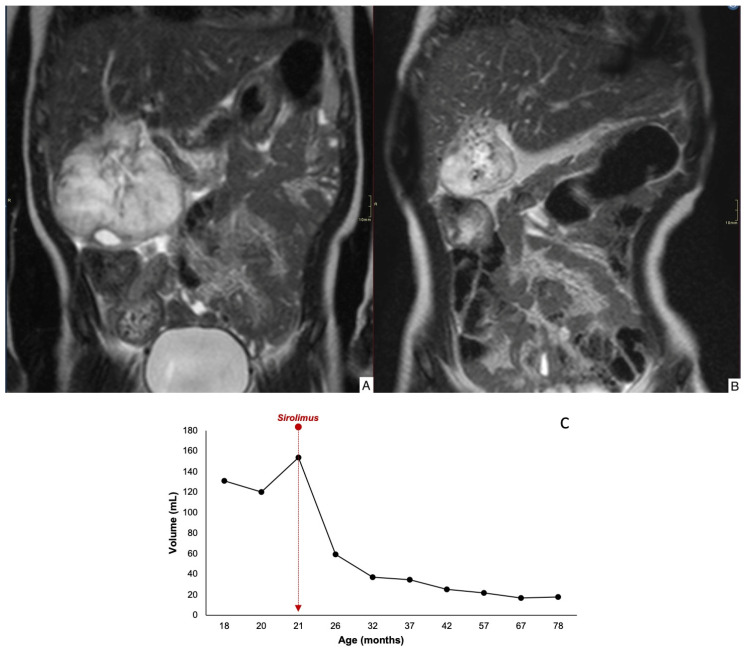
Coronal T2-weighted MRI image (T2WI) through the abdomen of an 1-yr-old girl depicts an exophytic and T2-hyperintense mass in the liver (A). Coronal T2WI after 18 months of treatment with sirolimus, the mass has markedly decreased in size (84% tumor volume reduction) (B). Time evolution curve of tumor volume (C).
